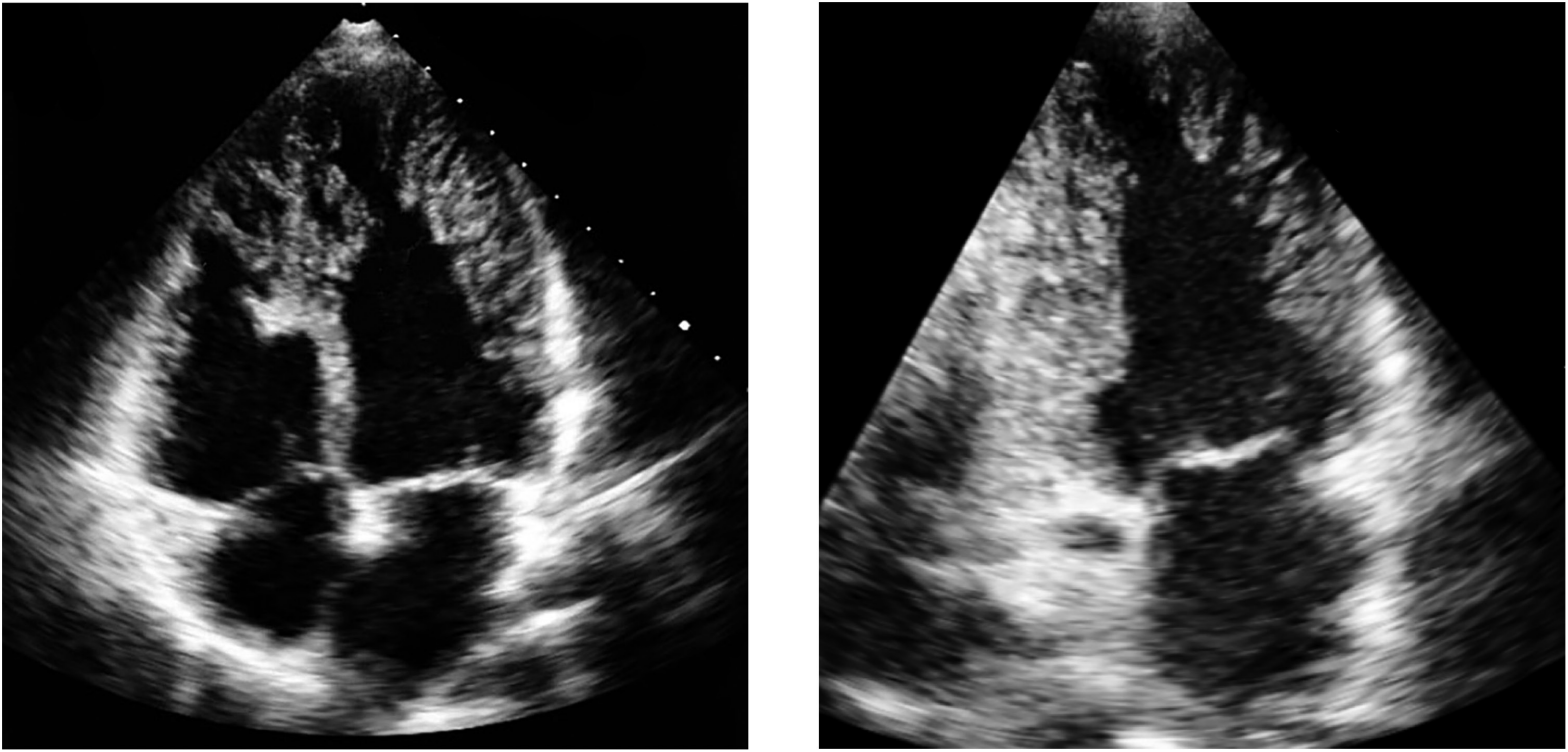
Apical four-chamber and two-chamber views on echocardiography demonstrating prominent trabeculations in both ventricles with deep intertrabecular recesses.
